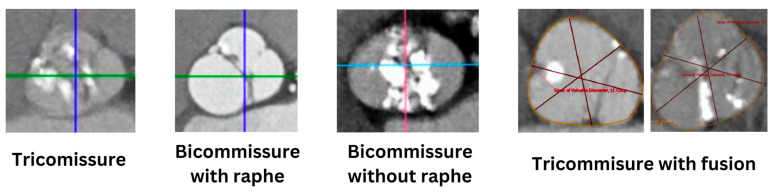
BAV classification in the TAVI era [7]. Images are derived from Computed Tomography scans. Blue lines at the first two images and pink line at the third image represent the vertical axis. Green lines at the first two images and light blue line at the third image represent the horizontal axis. The burgundy colored lines at the last two images were used to indicate the different dimensions during measurements.
